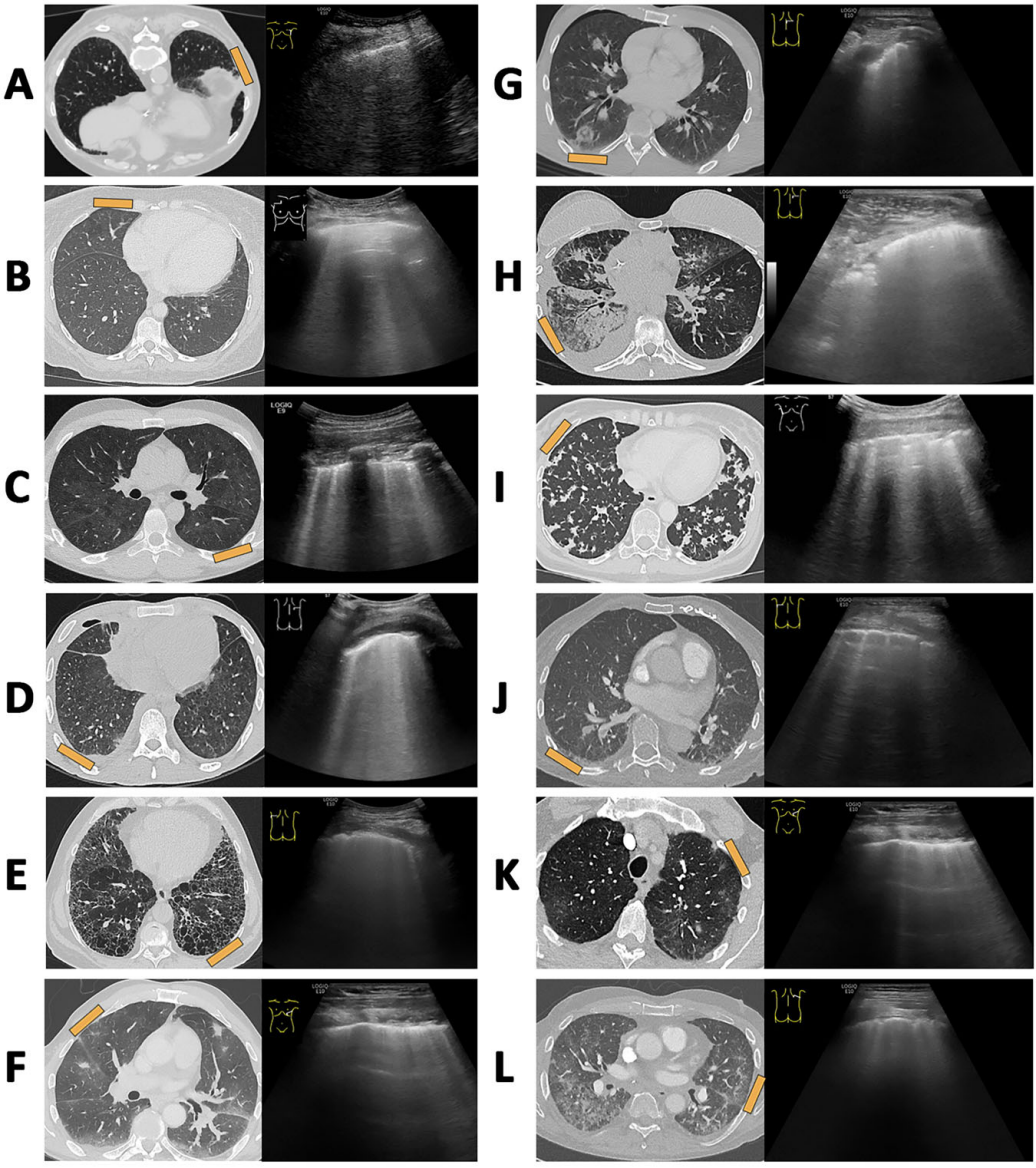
Different underlying causes of artifacts oriented vertically to the transducer in LUS. The orange marker on the CT scan (left) indicates the ultrasound probe position. A Patient with a history of smoking and changes caused by scarring. B Patient with cardiogenic pulmonary edema. C Patient with diffuse Pneumocystis jirovecii pneumonia. D Patient with pulmonary involvement in tuberculosis. E Patient with pulmonary fibrosis exhibiting a typical interstitial pneumonia pattern. F Patient with systemic sclerosis and pulmonary involvement. G Patient with lung contusion due to trauma. H Patient with breast cancer and lymphangitic carcinomatosis. I Patient with lepidic- to alveolar-growing carcinoma. J Patient with segmental pulmonary embolism. K Patient with pulmonary involvement in amyloidosis. L Patient with lung transplant rejection
